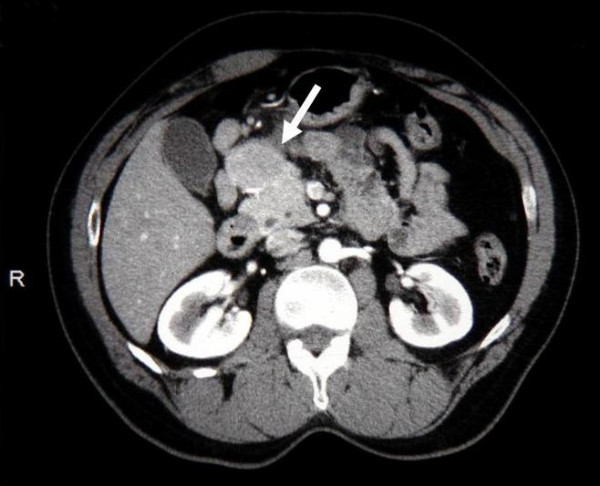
Computed tomography demonstrating enhancing mass protruding from the head of the pancreas. White arrow points to Castleman's disease in pancreas.
